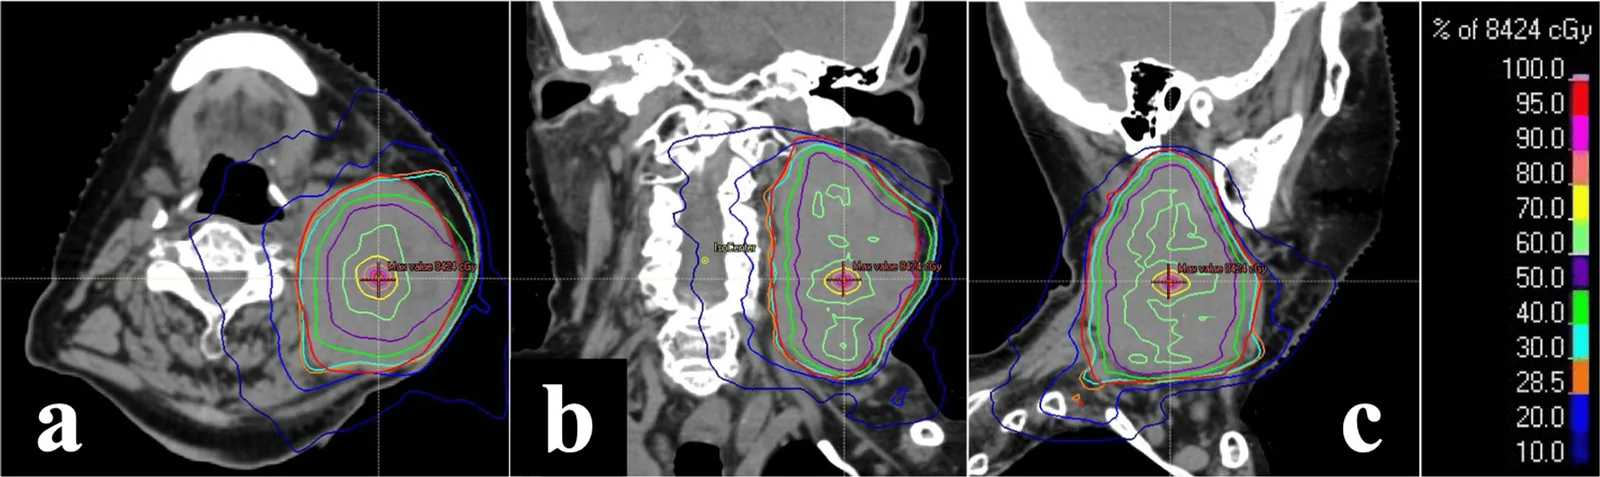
Initially planned dose distribution of SBRT Isodose lines are displayed relative to the maximum dose (100% = 84.24 Gy) on non-contrast CT. (a) Axial, (b) coronal, and (c) sagittal views. Irradiation time is 304 seconds per fraction via three arcs at O-ring angles of 0°, 20°, and 340° using the OXRAY® system (Hitachi High-Tech Corporation, Tokyo, Japan). SBRT; stereotactic body radiotherapy, CT; computed tomography.
